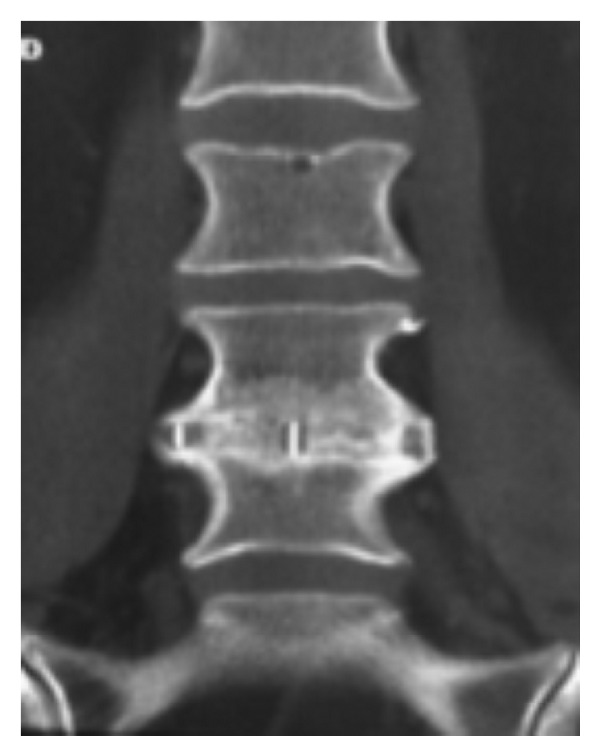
Coronal computed tomography (CT) showing solid arthrodesis at 12 months postoperative following L4-5 XLIF.
